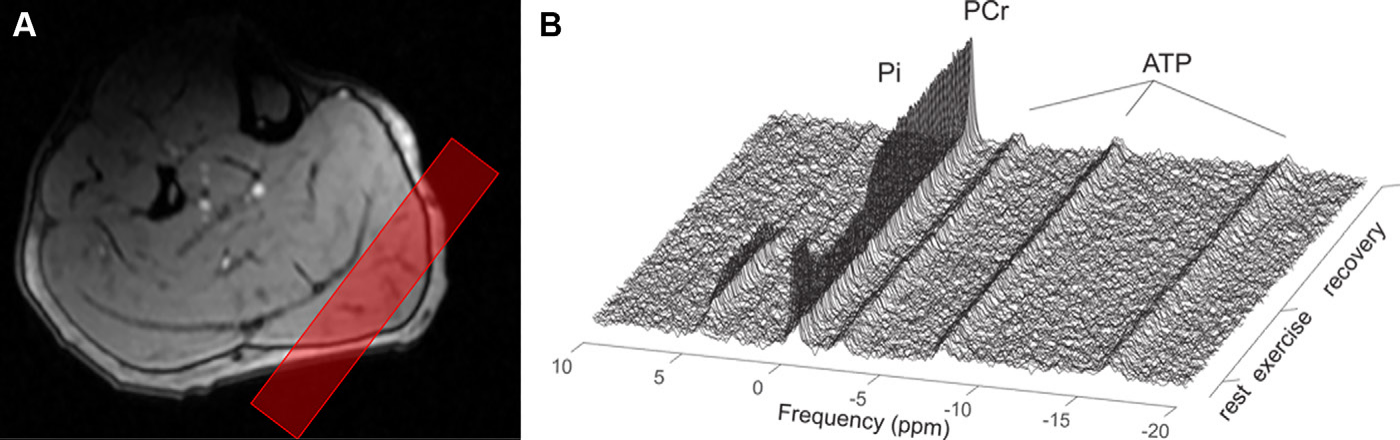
(A) Noncontrast MRI scan during ^31^P MR
